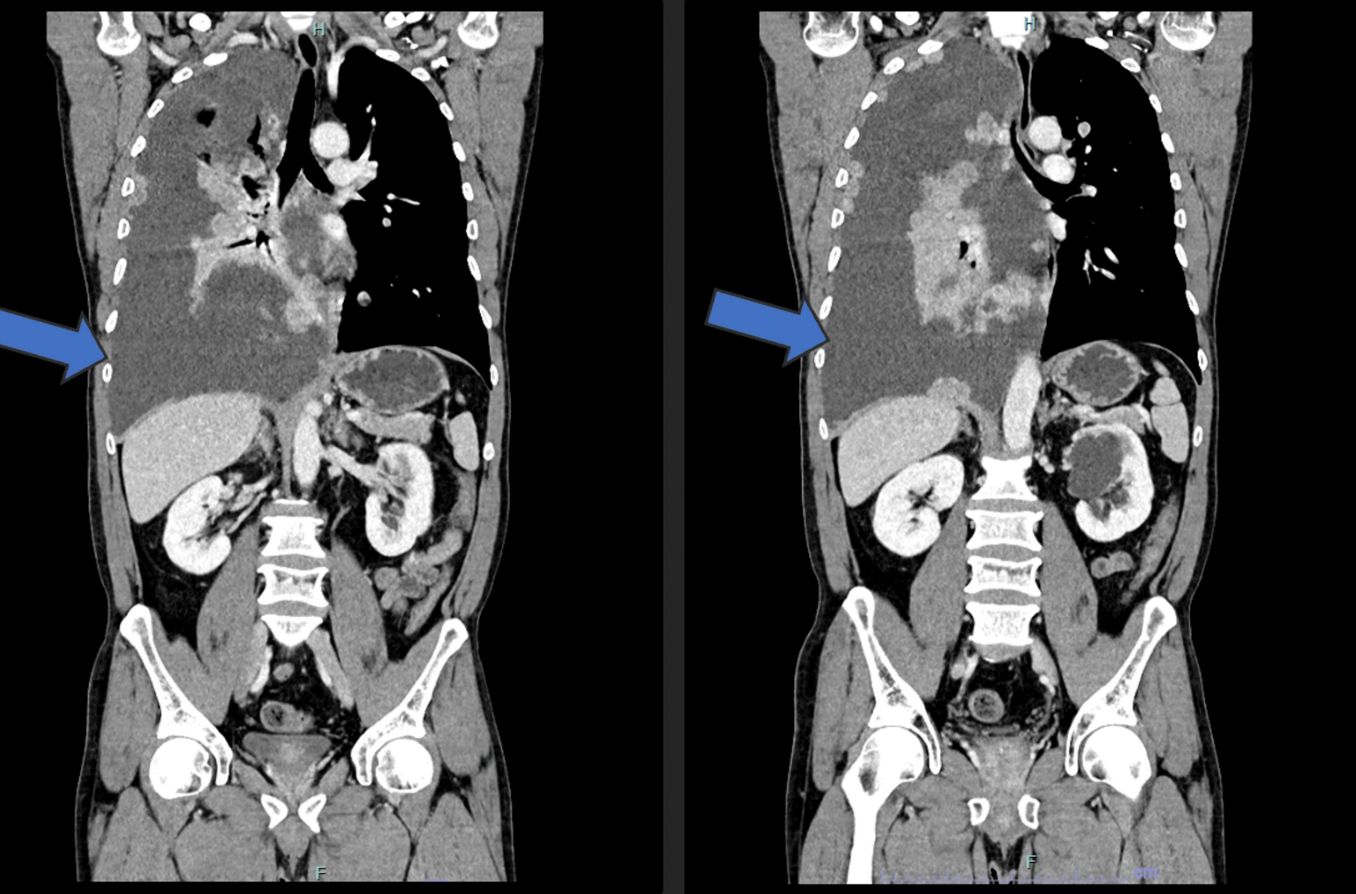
Thoracoabdominopelvic computed tomography (CT) showing metastatic disease.
